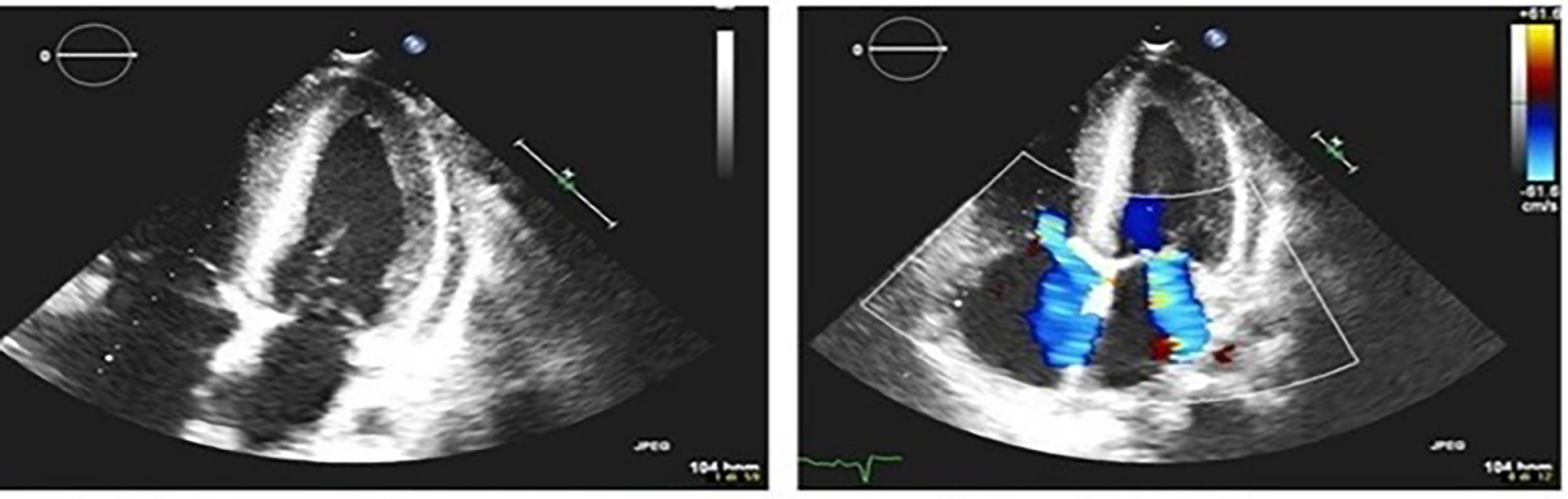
2D-echocardiogram shows moderate mitral regurgitation and thickening of the myocardium.
